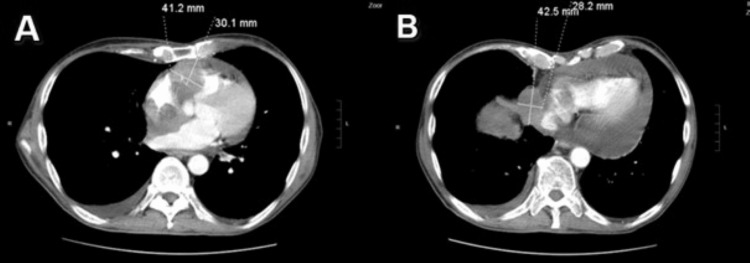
Chest CT (A) Showing 41.2 x 30.1-mm intracardiac mass causing indentation and marked mass effect on the superior and anterior aspect of the right ventricle. (B) Right pericardial mass measuring 4.2 x 2.8 cm abutting the right lateral aspect of the right ventricle and the junction of the SVC CT: computed tomography; SVC: superior vena cava
